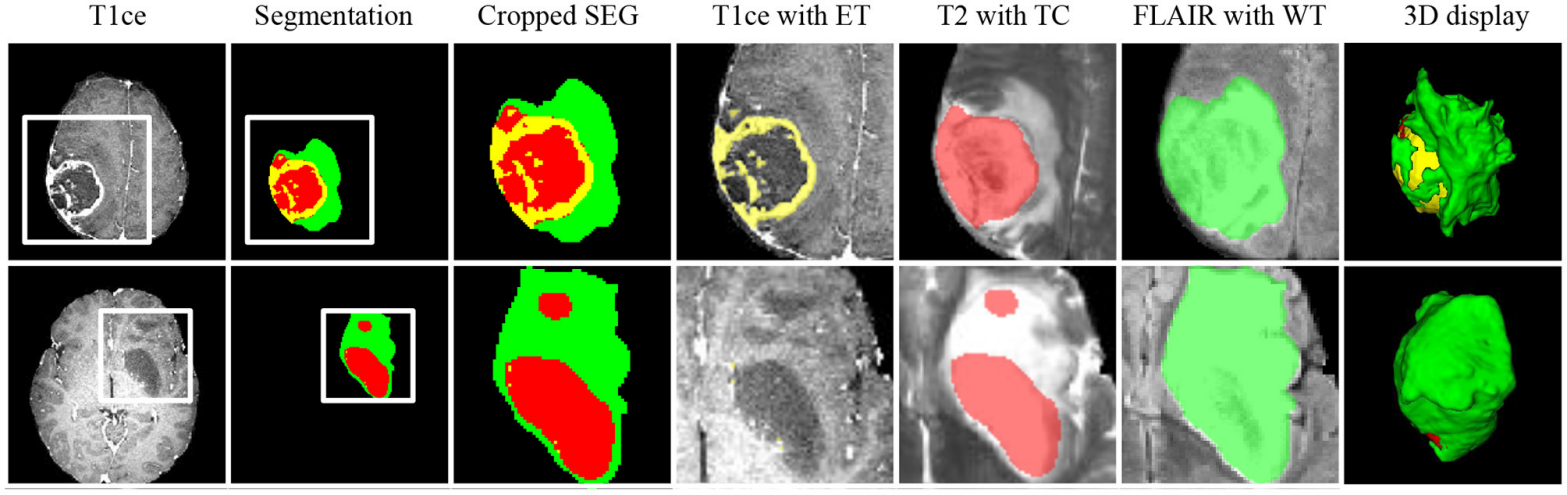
Representative cases from our in-house dataset of the two grades of tumors (high grade and low grade). From left to right: T1ce, automated tumor segmentation, zoomed-in segmentation, T1ce overlaid with ET, T2 overlaid with TC, FLAIR overlaid with WT, 3d display of tumor segmentation. ET, enhancing tumor; TC, tumor core; WT, whole tumor.
